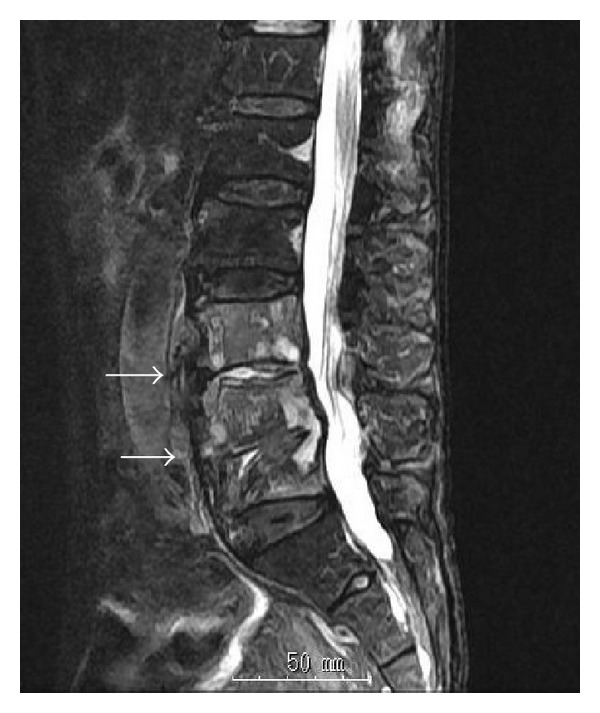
MRI showed the collapse of L5 and an increased signal intensity in the L3, 4, 5 vertebral body on T2 STIR images (arrow).
